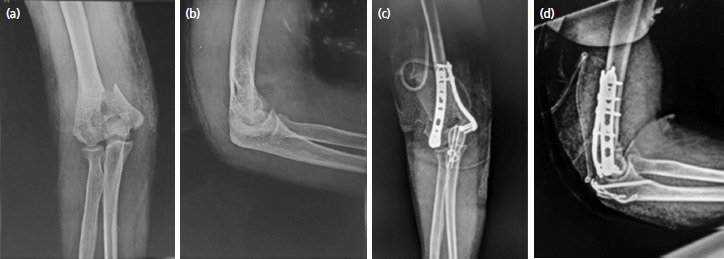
(a) Pre-operative radiograph of elbow after Gigli saw in AP and (b) lateral view of a 54-year-old female, a post-traumatic case of right sided distal humerus intra-articular fracture closed with no distal neurovascular deficit; (c) Post-operative radiograph of elbow in AP and (d) lateral view of a 54-year-old female, a post-traumatic case of right sided distal humerus intra-articular fracture closed with no distal neurovascular deficit.
